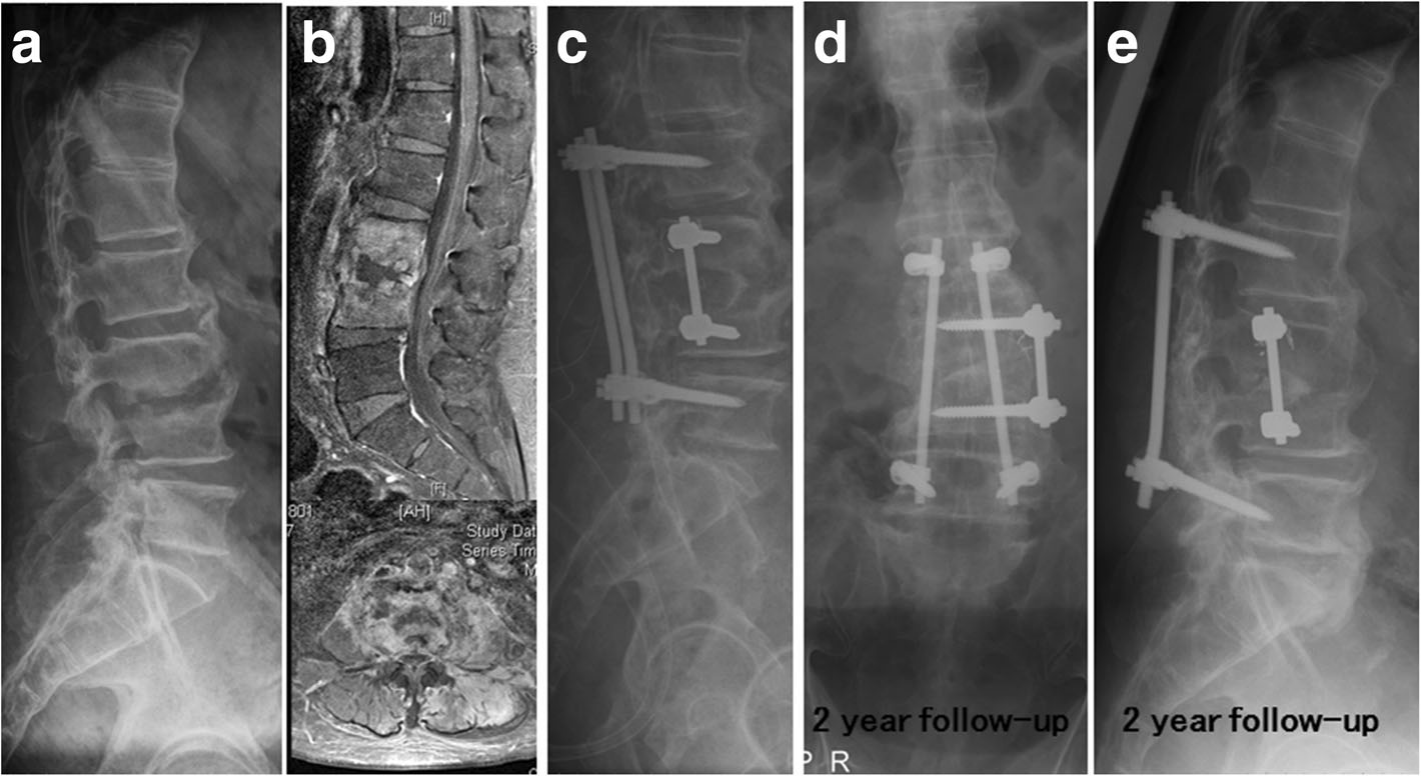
Escherichia coli infectious spondylodiscitis of L3-4 in a patient with a past history of colon cancer (a). Disc space was narrowing with nearby destructed endplates on L3-4 level; b Osteomyelitis in L3-4 vertebral bodies, discitis and psoas abscess without epidural abscess were found in gadolinium-enhanced magnetic resonance imaging; c Anterior and posterior spinal surgery was noticed in the immediate postoperative X-ray.; d and e Solid bone fusion was noticed at the 2-year follow-up
